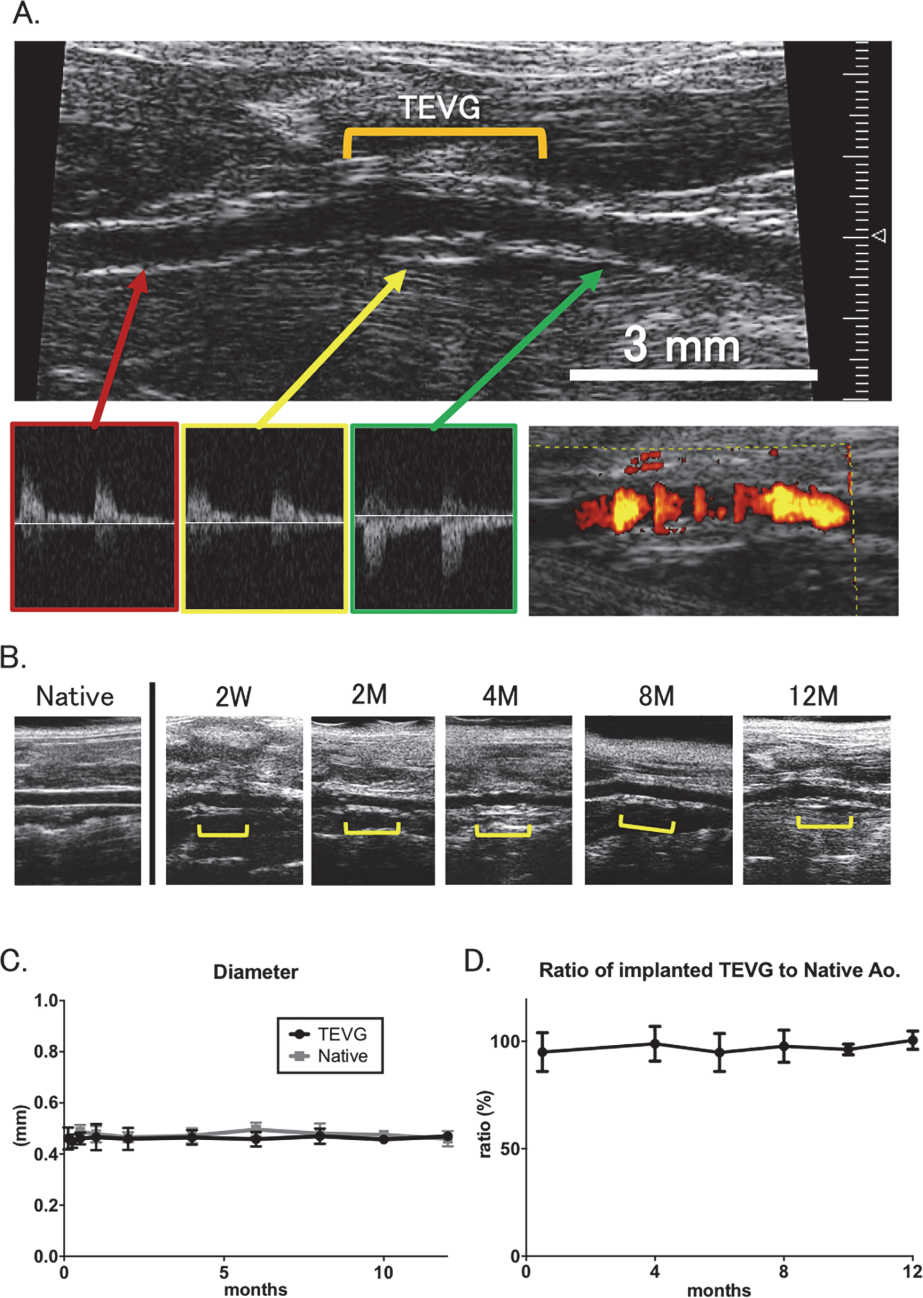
Luminal patency and laminar flow were similar between TEVG and native aorta over the course of the 12 month experiment. A. Doppler ultrasound examinations were performed on tissue engineered vascular grafts from 2 weeks to 12 months after implantation. Doppler signals proximal to the graft, distal, and within the graft showed propagation of systemic arterial impulse through the graft. B.
